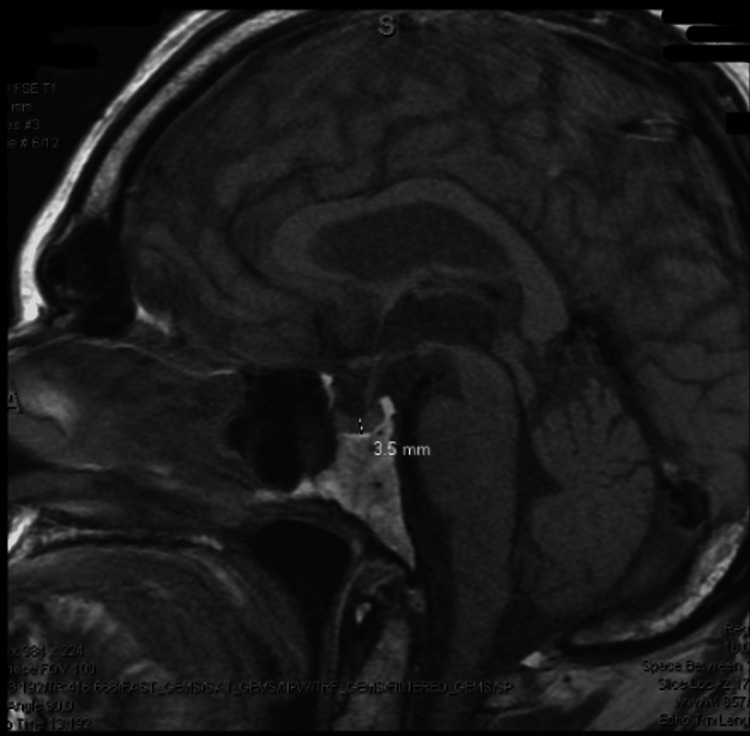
MRI of brain with contrast. The MRI shows the unremarkable appearance of the sella and pituitary gland, which measures approximately 3.5 mm in the cranial and caudal dimensions.
